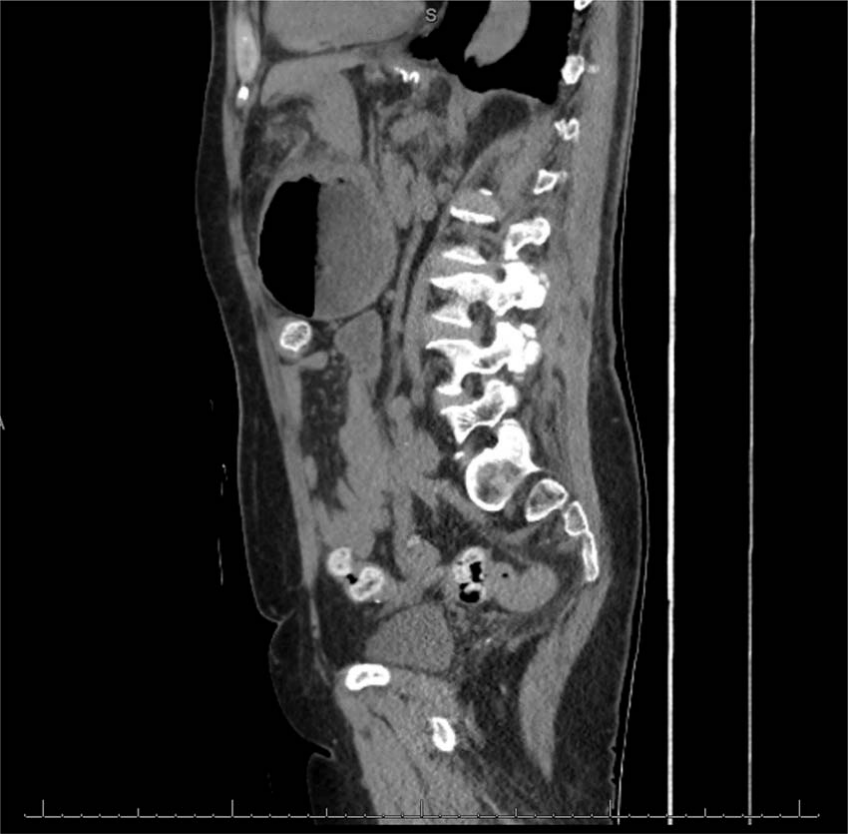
Sagittal view of CT scan of abdomen and pelvis demonstrating gastric volvulus.
